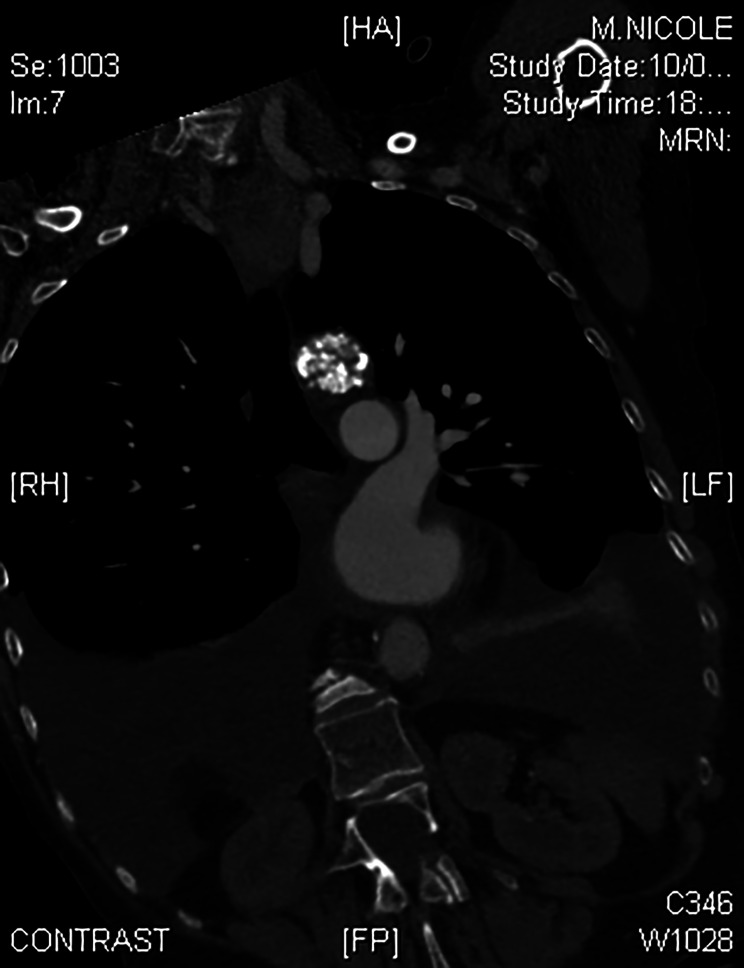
CT scan (frontal cut) showing massive calcifications in the aortic isthmus
